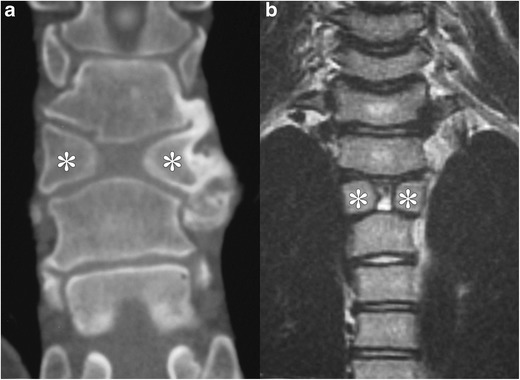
Butterfly vertebra in two patients: Coronal reformatted image from a non-contrast CT of the spine (*a*) and coronal T1-weighted MR image of the upper thoracic spine (*b*) both demonstrate separate ossification centres on each side of the vertebral body (marked by *asterisks*). Note that there is moulding of the adjacent vertebral bodies towards the midsagittal constriction, especially pronounced on a
